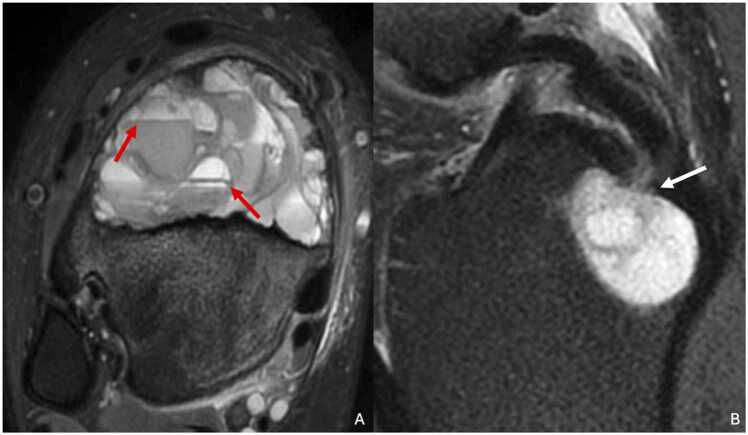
A. Aneurysmal bone cyst of the distal metaphysis of the right tibia in a 15-year-old male adolescent with pain persistent after a sprain. B. Intraosseous mucoid cyst of the proximal epiphysis of the right tibia in a 43-year-old man (different patient). A. T2-weighted fat-suppressed MR image in the axial plane showing a large heterogenous lesion with liquid-liquid levels (red arrows). B. T2-weighted fat-suppressed MR image in the sagittal plane showing a 2 cm cystic lesion of the proximal epiphysis communicating with the insertion of the posterior cruciate ligament (white arrow).
